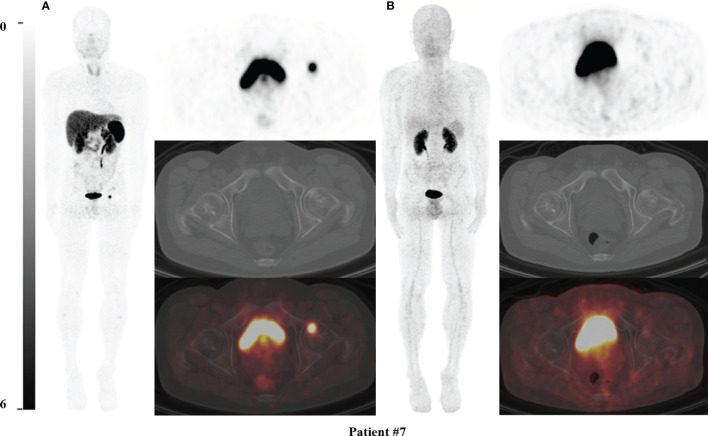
^68^Ga-DOTA-TATE PET/CT comparing with ^68^Ga-DOTA-JR11 PET/CT in Patient #7. The causative tumors of TIO were found in the left femoral head in patient #7 (A) on ^68^Ga-DOTA-TATE PET/CT, but negative on ^68^Ga-DOTA-JR11 PET/CT (B). The lesion of the left femoral head was confirmed as the causative tumor of TIO by postsurgical pathological results.
